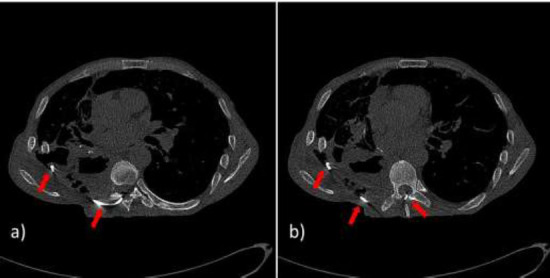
Axial view of thoracic CT showing titanium costa material protruding into the vertebral epidural space. In these sections, air in the dural area could be seen too.
